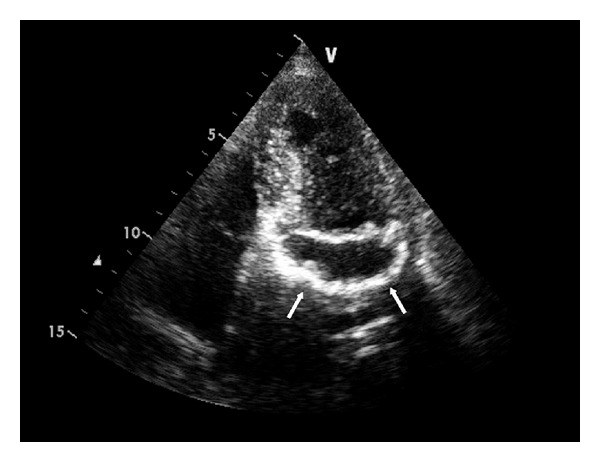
Echocardiographic apical four-chamber view showing a large oval echolucent mass with echodense rim in the mitral annular region.
